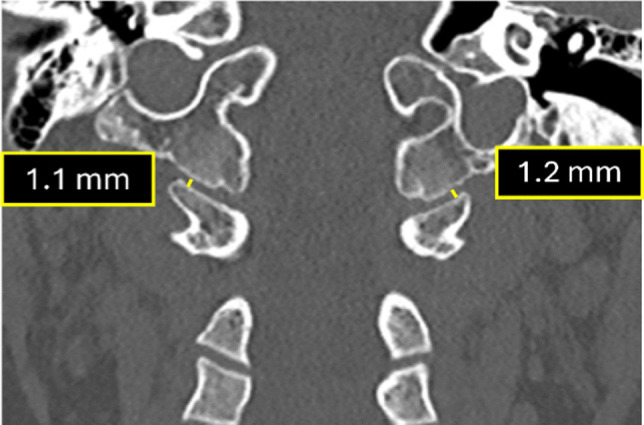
Coronal CT demonstrating normal occipital condyle–C1 interval measurements. The minimum joint space is measured between the occipital condyle and the C1 lateral mass along the lateral aspect of the joint, with exclusion of the medial occipital notch to avoid false-positive interpretation (normal <3.5 mm)
